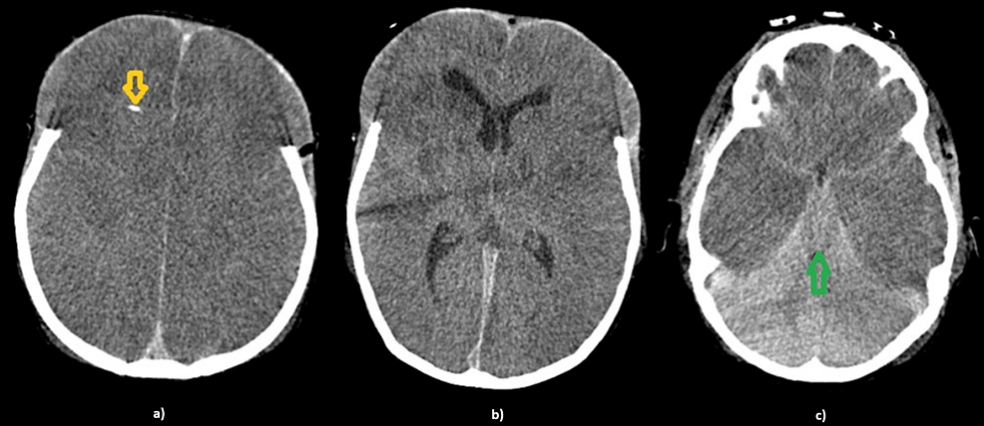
Head computed tomography imaging revealing pronounced global hypodensity in both cerebral hemispheres, absence of grey and white matter differentiation and complete effacement of the extra-ventricular supratentorial spaces. The brainstem mass effect is more exuberant, with a notable mesencephalic compression due to bilateral uncal herniation (green arrow on c). There is also a greater conflict of space in the posterior fossa. Brain parenchyma is herniated through the site of the bilateral frontal craniectomy. An intracranial pressure monitoring catheter (yellow arrow on a) is placed in the anterior horn of the right lateral ventricle.
